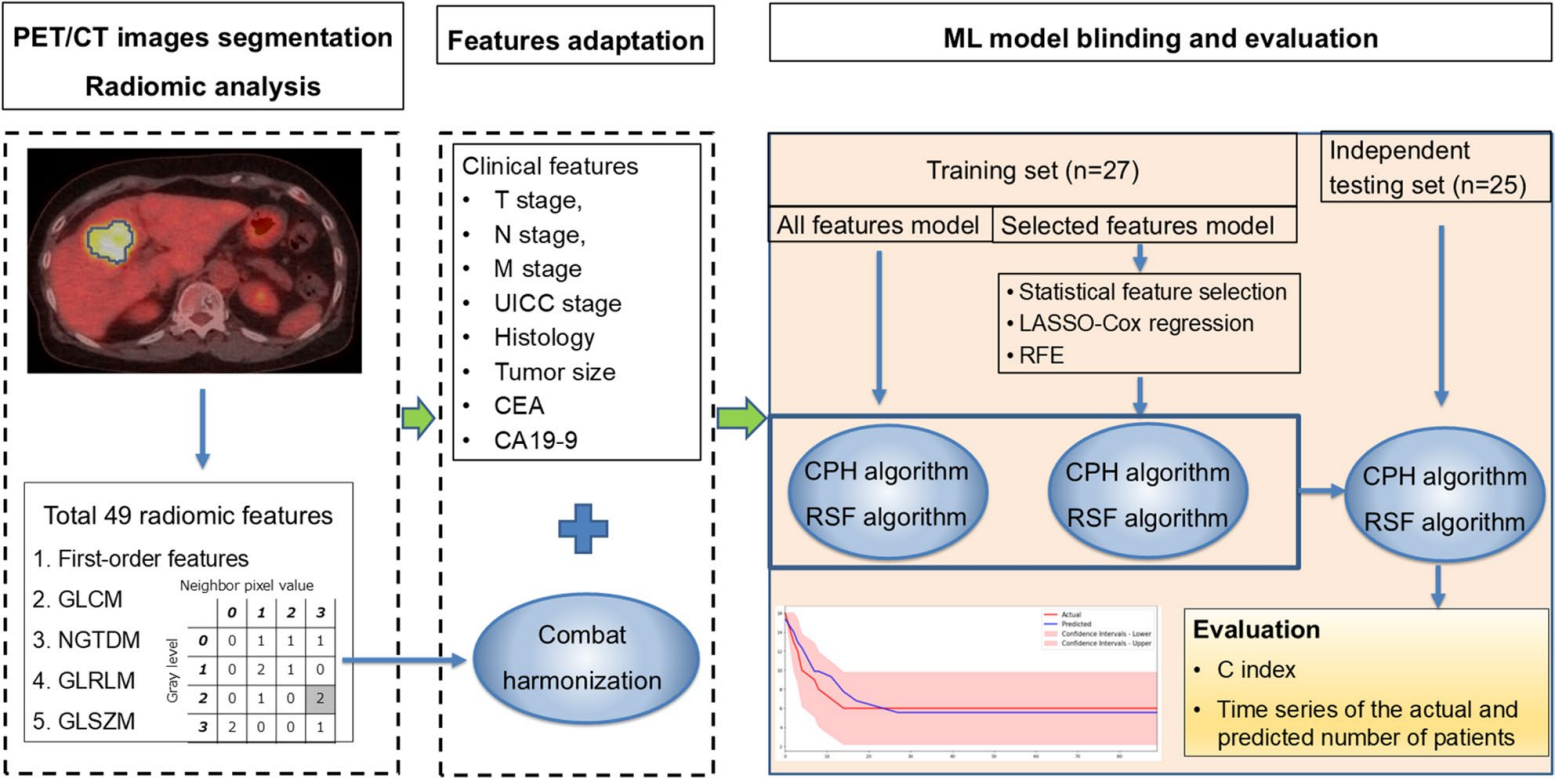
Work flow of the present study. Radiomics features have been extracted from the volume of interest segmented from PET/CT images. Then ComBat harmonization is applied to each radiomic feature due to two different PET scanners. Finally, two ML models (all features ML model and selected features ML model) are developed to predict PFS of the patients with gallbladder cancer using clinical features and PET/CT radiomic features
